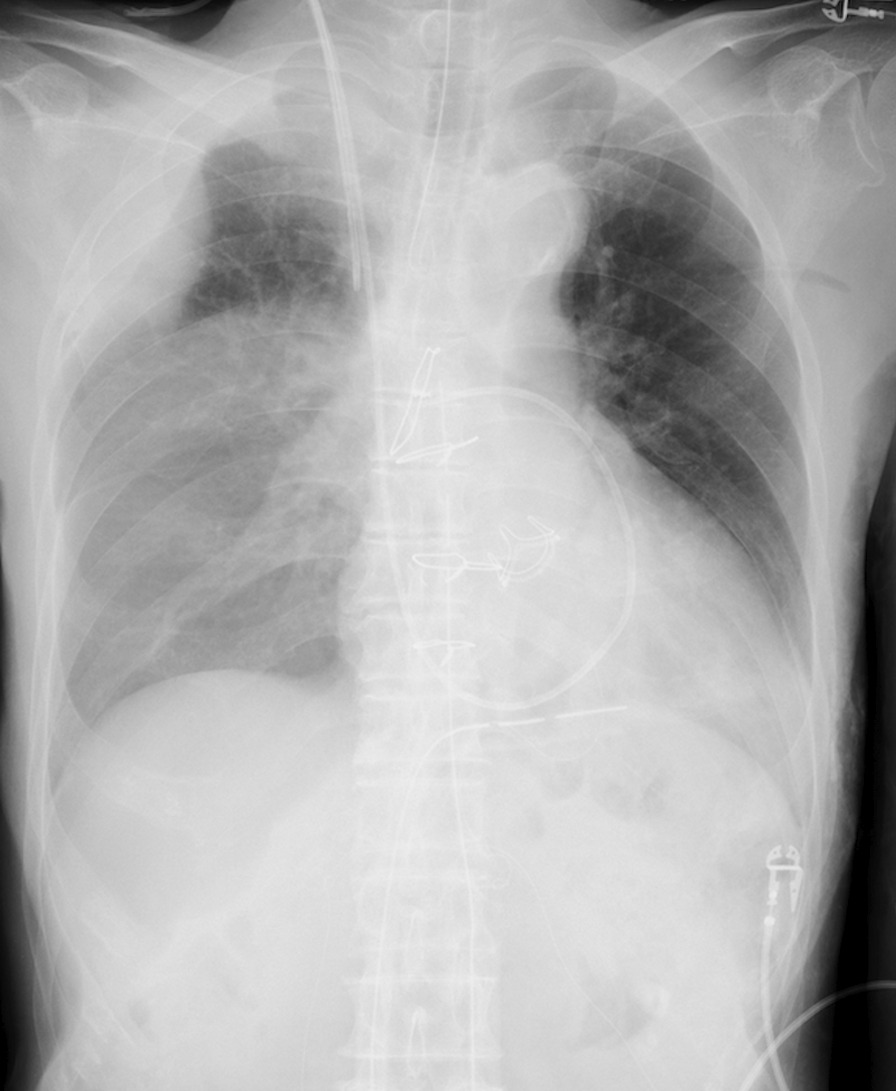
Postoperative chest radiograph showed a hematoma on the right upper chest wall and hemothorax
